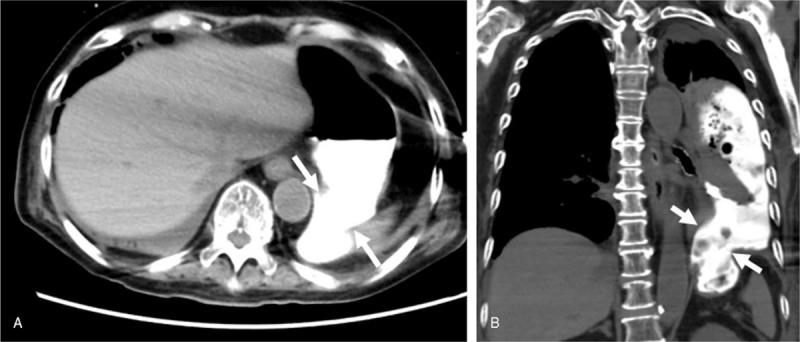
Chest computed tomography after administration of diluted amidotrizoate in the coronal (A) and transverse (B) planes. White arrows indicate the gastropleural fistula.
